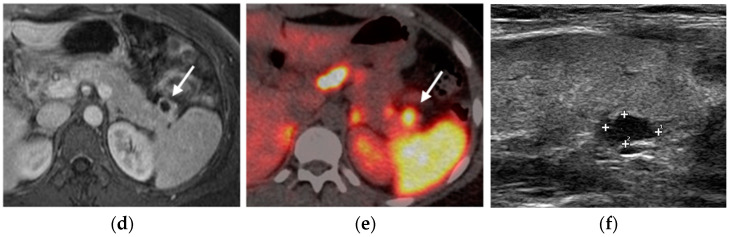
Cystic pancreatic NET of the tail (arrows). Fat-sat T2-weighted image (a) and T1-weighted image (b) show a cystic-like lesion in the pancreatic tail with a thick and irregular enhancing wall (c,d). PET-CT highlights high tracer uptake of the lesion (e). In the same patient, ultrasound revealed a synchronous parathyroid adenoma (f).
